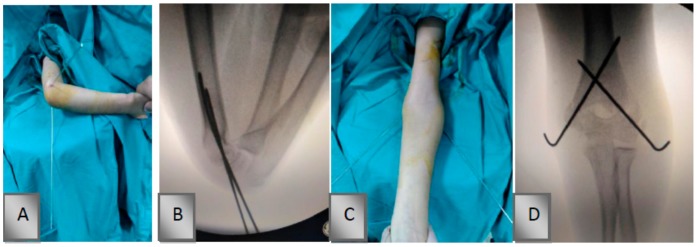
Crossed Kirschner (K)-wire configuration and its fluoroscopic image. (A,C) Anteroposterior and lateral positions after K-wire configuration and (B,D) intraoperative radiograph with crossed K-wire configuration.
